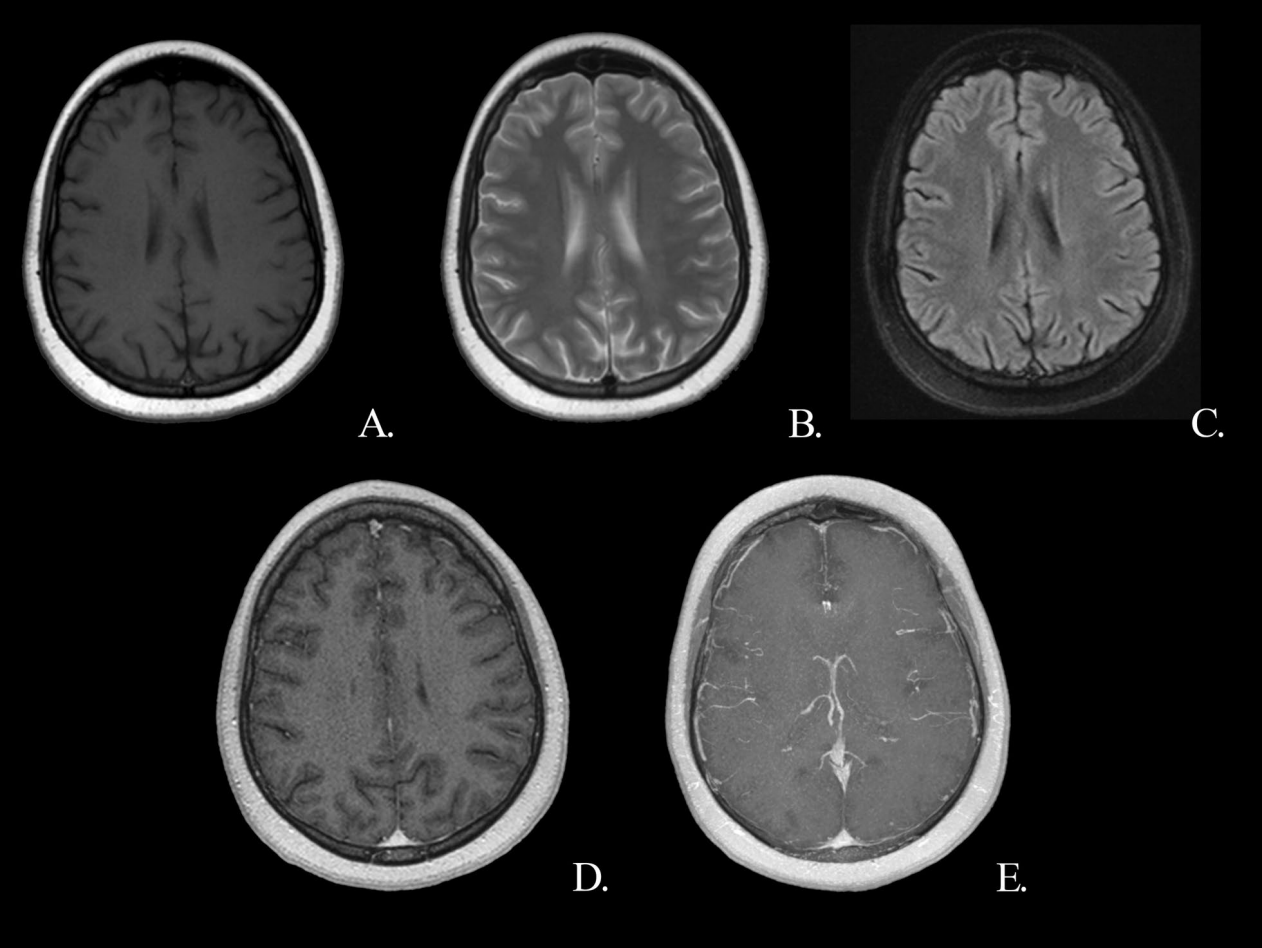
Control MRI after 2 months of anti-tuberculosis theraphy demonstrates resolution of the lesions. Precontrast T1W axial (A), T2W axial (B), FLAIR axial (C), post-contrast T1W axial (D), post-contrast T1W axial MIP images (E). *FLAIR* fluid-attenuated inversion recovery, *MIP* maximum intensity projection, *T1W* T1 weighted, *T2W* T2 weighted
